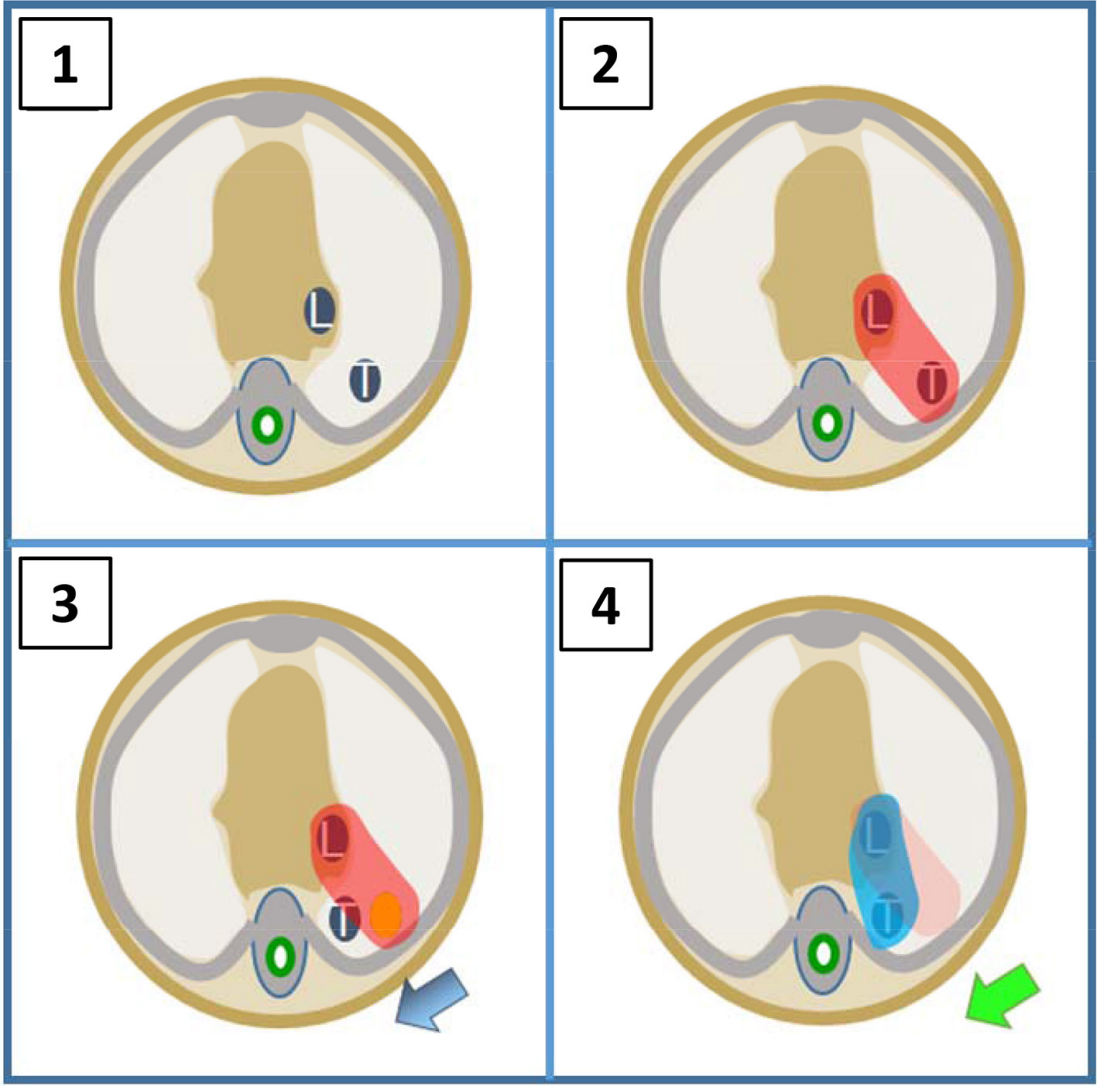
Treatment adaptation. (1) RTP CT demonstrates locally advanced lung cancer, tumour (T) and lymph node (L). (2) RTP CT with PTV covering T and L. (3) On-treatment image shows baseline shift causing tumour to move outside of PTV and high risk of geographical miss. (4) Treatment adapted to produce new PTV covering new position of L and T. PTV, planning target volume; RTP, radiotherapy treatment planning.
